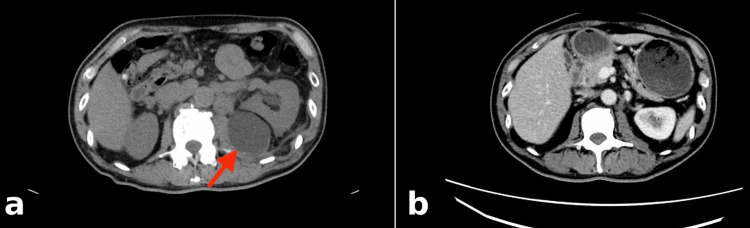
CT images of the retroperitoneal mass before and after degarelix treatment. (a) Metastatic mass before the initiation of degarelix therapy (red arrow). (b) Four weeks after the initiation of degarelix, the mass demonstrates a marked reduction in size. CT: computed tomography
